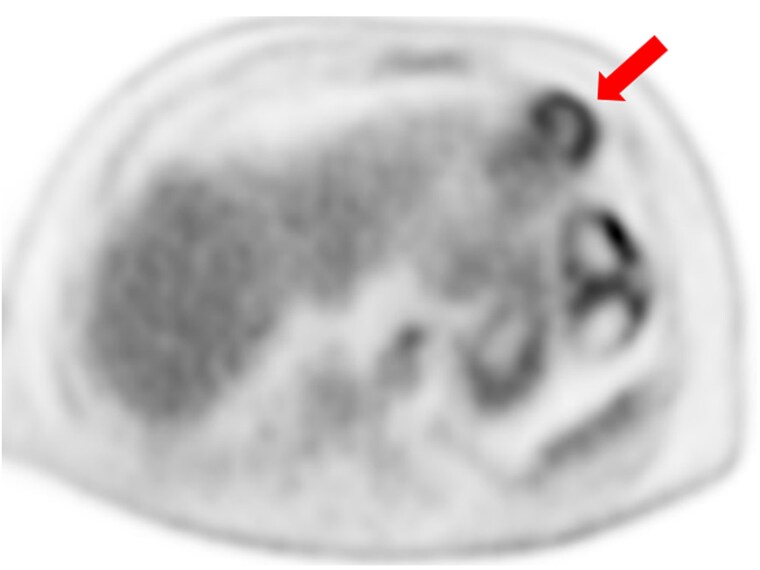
Axial PET image showing focal FDG uptake at the left ventricular apex with a central photopenic core, consistent with an avascular thrombus centre.
